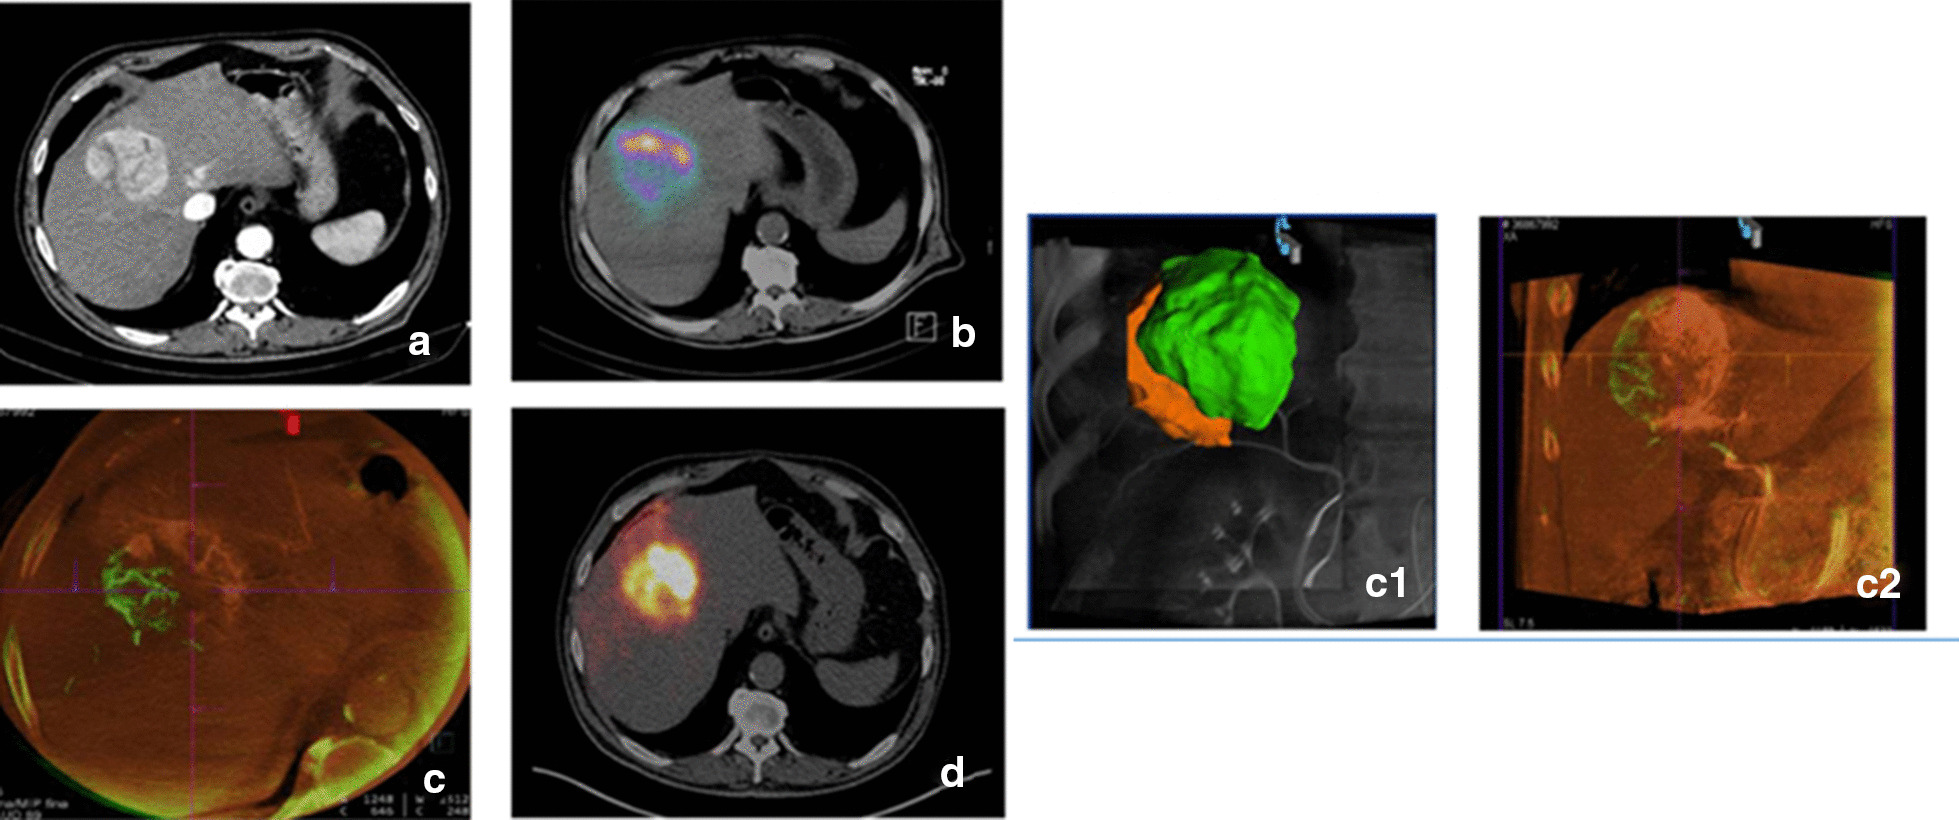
Same patient as Fig. 1. a Contrast-enhanced computed tomography (CECT) image: HCC located between segments IV and VIII. b
